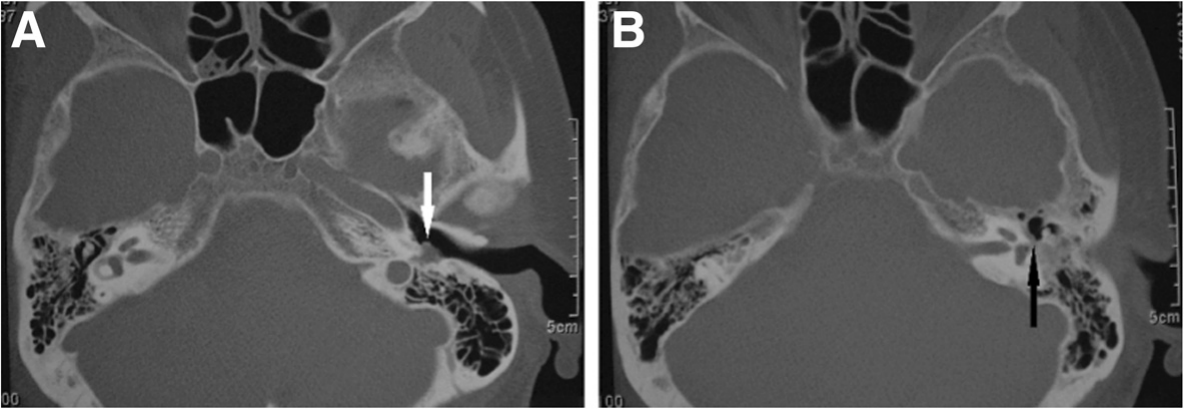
Axial computed tomography of the temporal bone shows a slightly ill-defined left middle ear soft tissue mass involving (A) the posterior mesotympanum *(white arrow)*, and (B) the posterior epitympanum and mastoid antrum with erosion of the facial fallopian canal around the second genu *(black arrow).*
